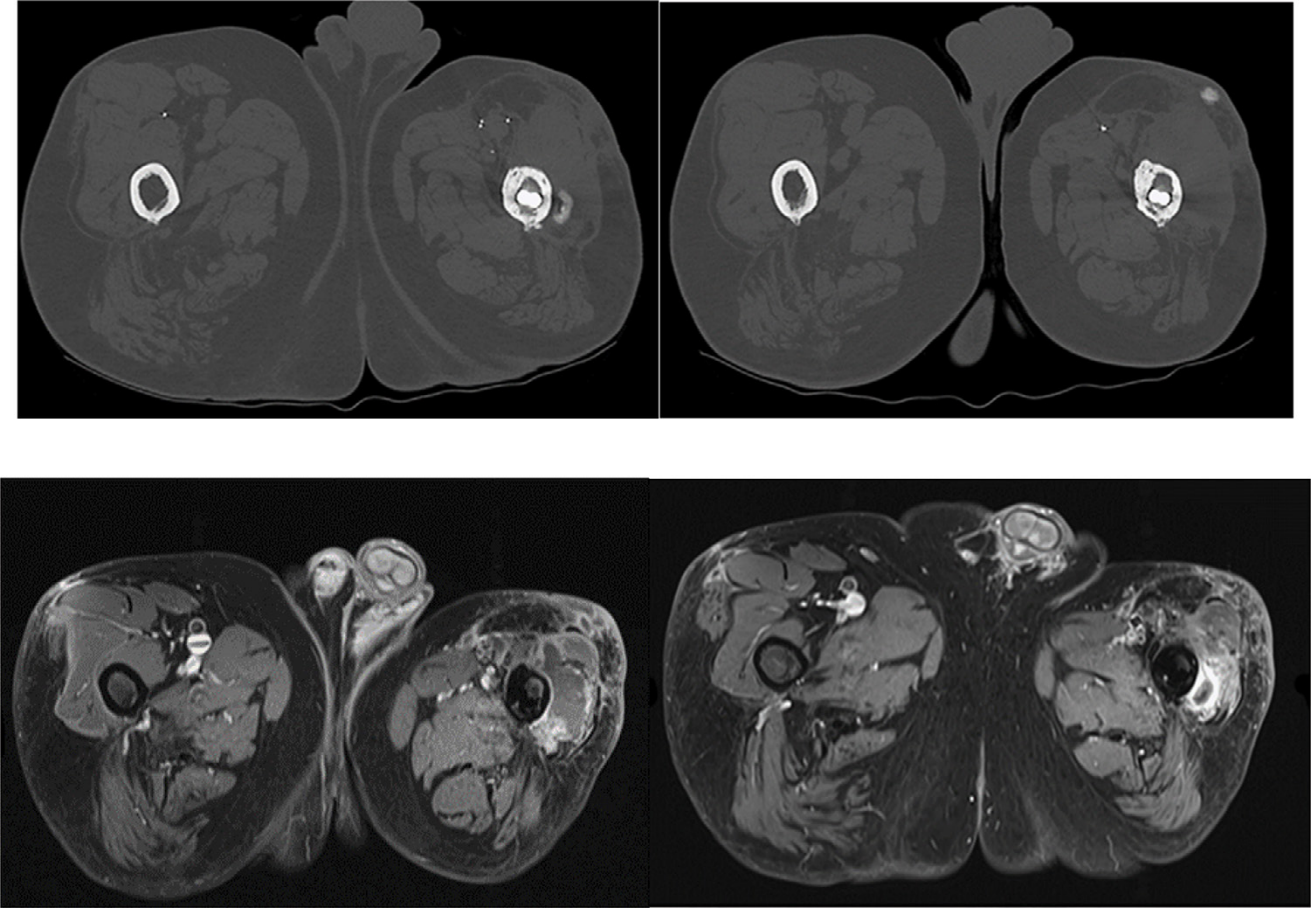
CT scan with bone window and MRI T1‑weighted fat‑saturated sequences after intravenous injection of gadolinium complex.
